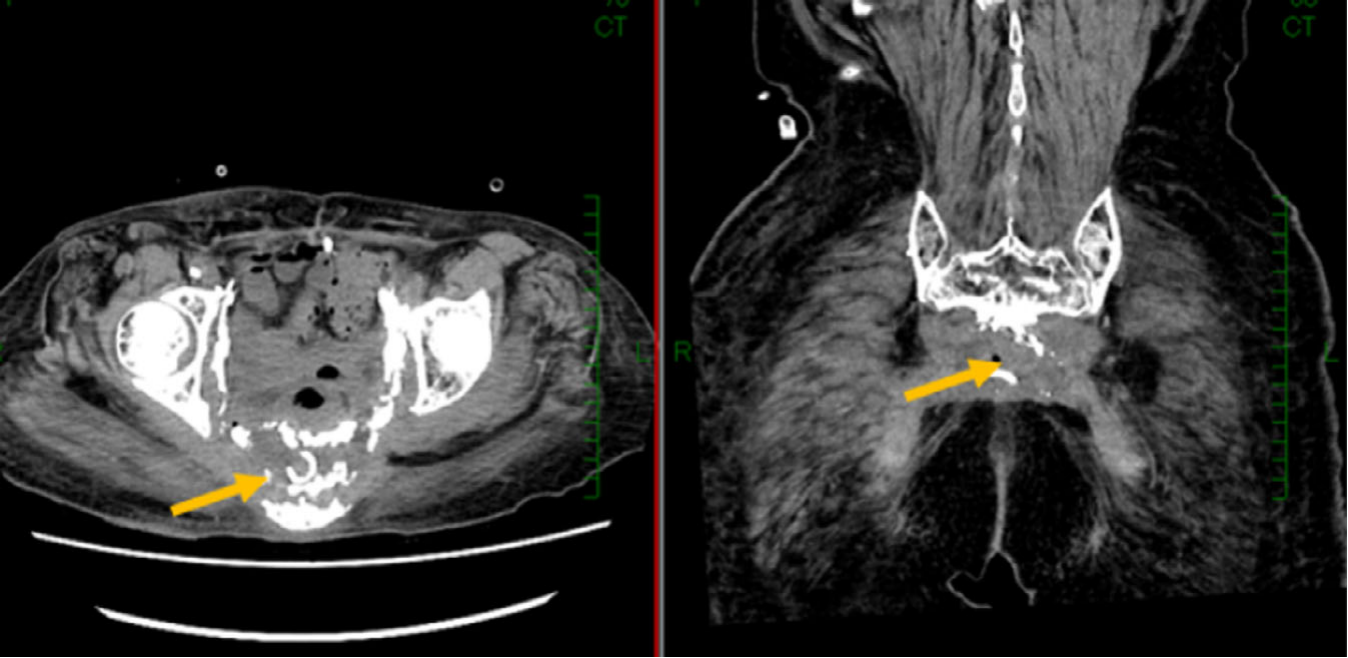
CT scans showing we performed CT‐scan guided drainage at the site of the retroperitoneal abscess identified in Figure 1a (yellow arrowheads).
